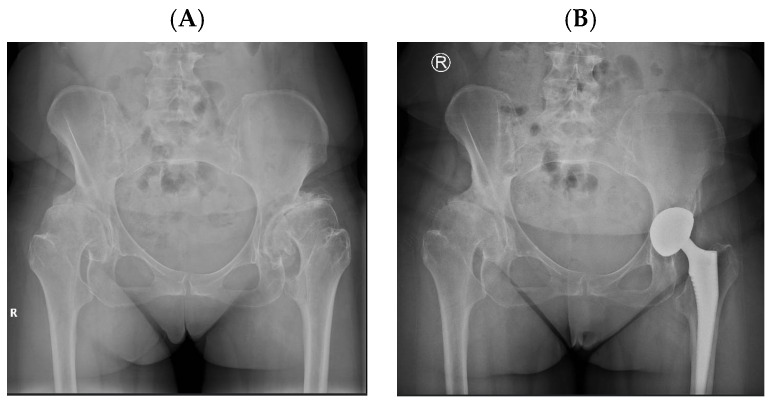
(A) Preoperative anteroposterior pelvic radiograph showing advanced hip osteoarthritis secondary to developmental dysplasia. (B) Postoperative radiograph following THA with a DM acetabular component.
